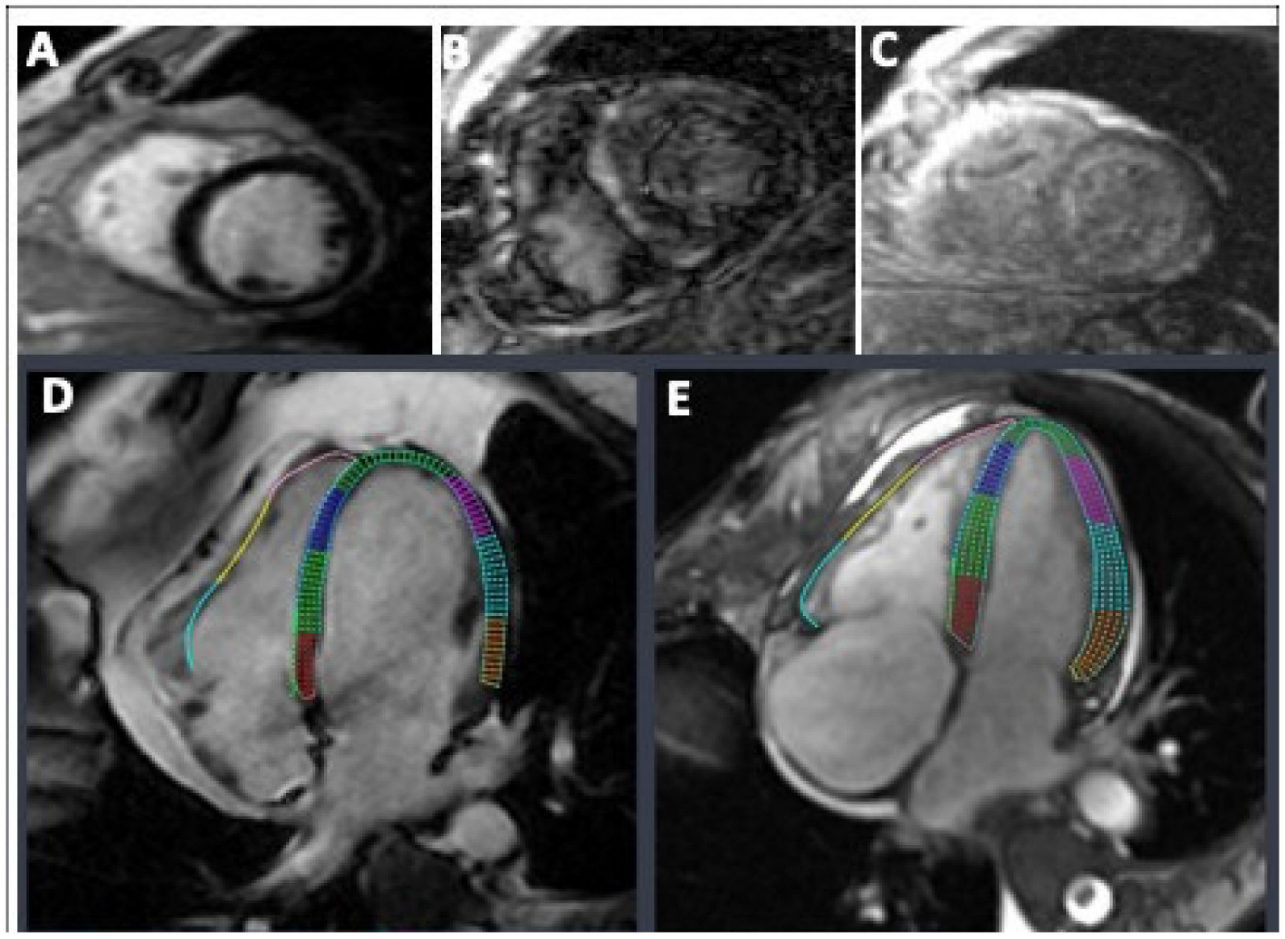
Cardiac MRI (CMR) visualization of cardiac amyloidosis using late gadolinium enhancement (LGE) and strain imaging. (A–C) Lack of myocardial nulling and characteristic speckled appearance are noted after gadolinium injection in cardiac amyloidosis. (A) Representative LGE-CMR short-axis image of a normal patient. (B) Representative LGE-CMR short-axis image of an ATTR amyloidosis patient. (C) Representative LGE-CMR short-axis image of an AL amyloidosis patient. (D,E) Visualization of left and right ventricular longitudinal strains under 4 chamber (4CH) long-axis view. (D) Representative 4CH-CMR image of a normal patient. (E) Representative 4CH-CMR image of an amyloidosis patient.
